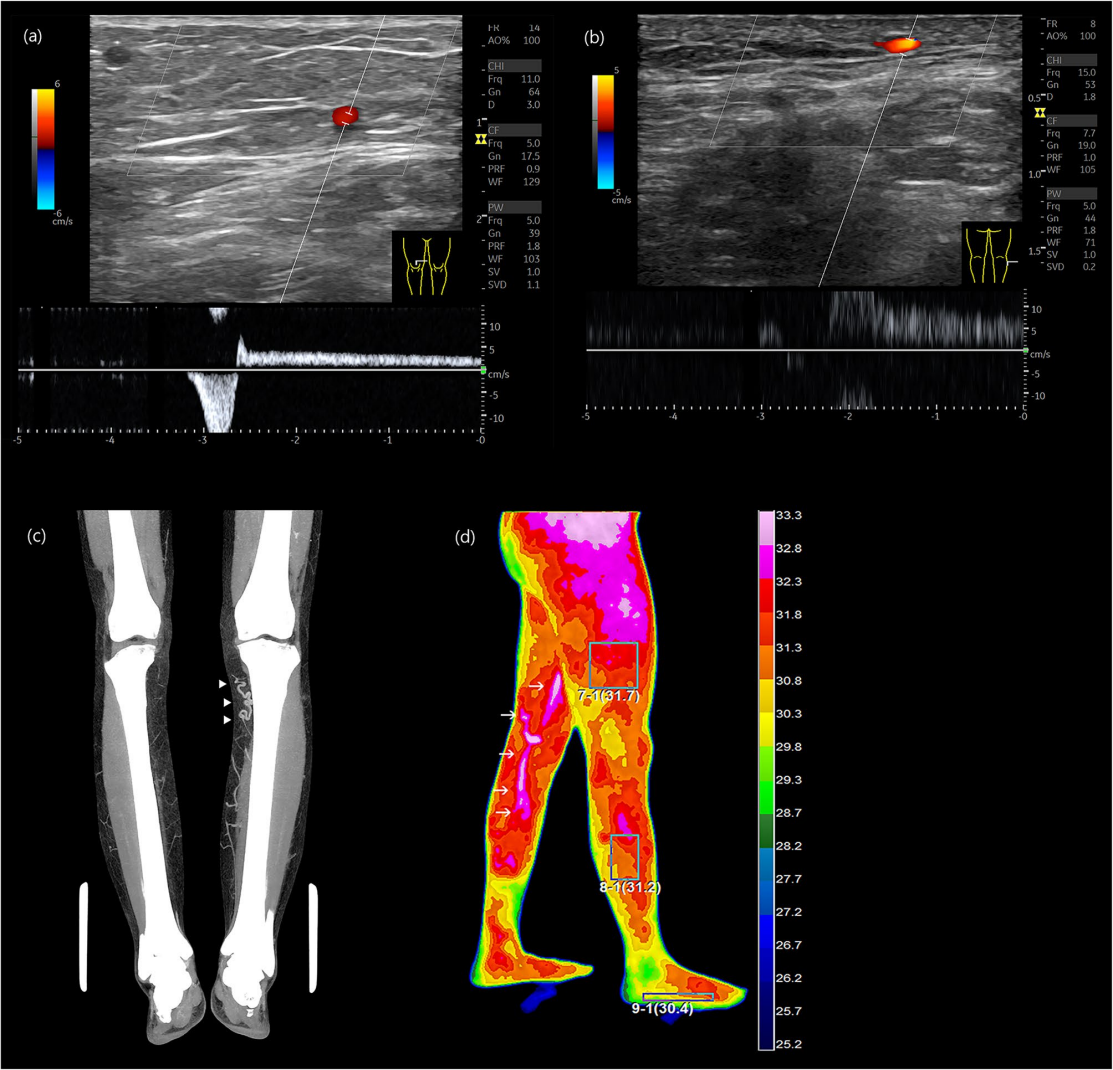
Diagnosis of CVD. (a) Axial ultrasound image showing GSV reflux at right lower thigh, (b) Axial ultrasound image displaying direct tributary reflux at right lateral calf, (c) Venous phase of lower extremity CT revealing a tortuous GSV at left upper calf indicating varicose change (arrowhead), (d) DITI showing GSV reflux at left medial thigh and calf (arrow).
